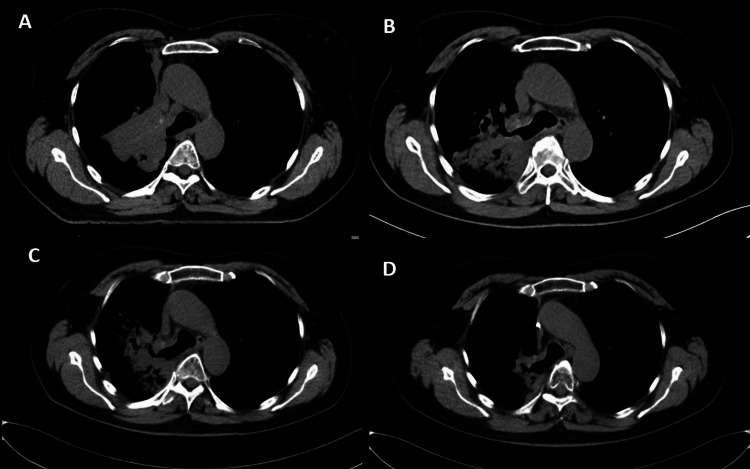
Chest CT scans before (A) and after (B,C,D) radiation therapy (A) Before treatment; (B) two months after RT; (C) four months after RT; (D) 16 months after RT
